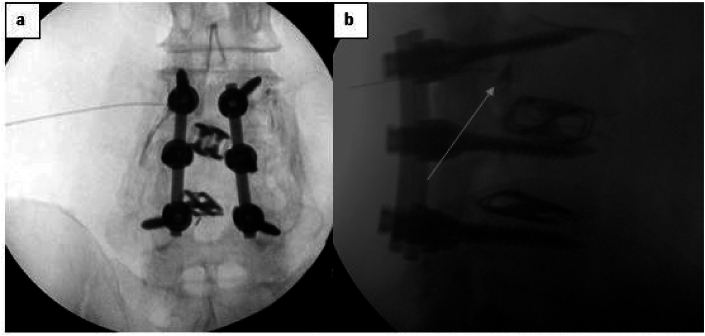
a) AP fluoroscopic radiograph depicting the radicular epidural flow of contrast in TFESI at level of previous laminectomy and fusion b) Lateral view of same procedure with arrow pointing at contrast flowing in the anterior epidural space.
